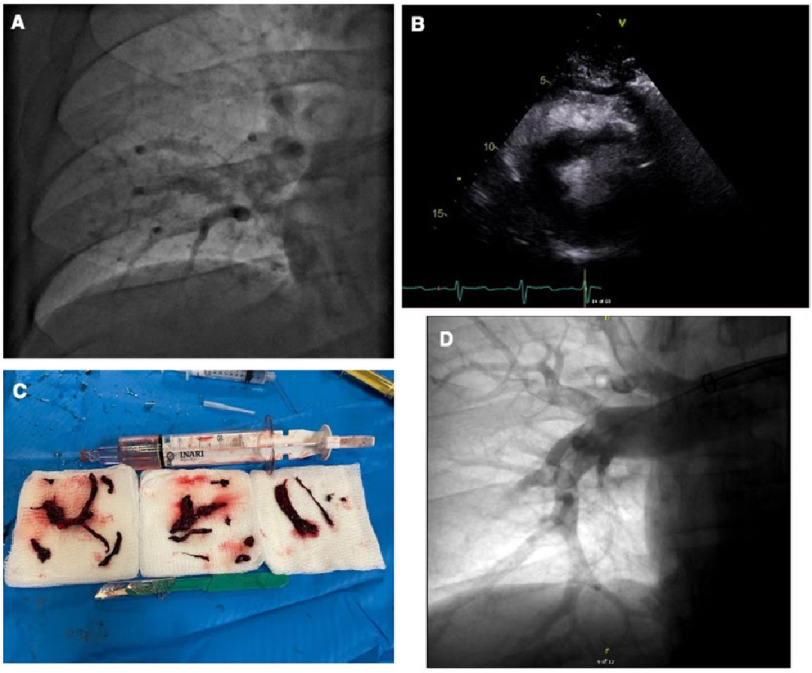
Pulmonary CT angiogram showing a large thrombus burden in the distal right main PA before thrombectomy (A). Right ventricular clot burden shown by transthoracic echocardiogram (B). The transthoracic echocardiogram shows large mobile thrombi in the right ventricle. Thrombus removed from the right PA with the Inari device. D: Final pulmonary angiogram after thrombectomy (C).
